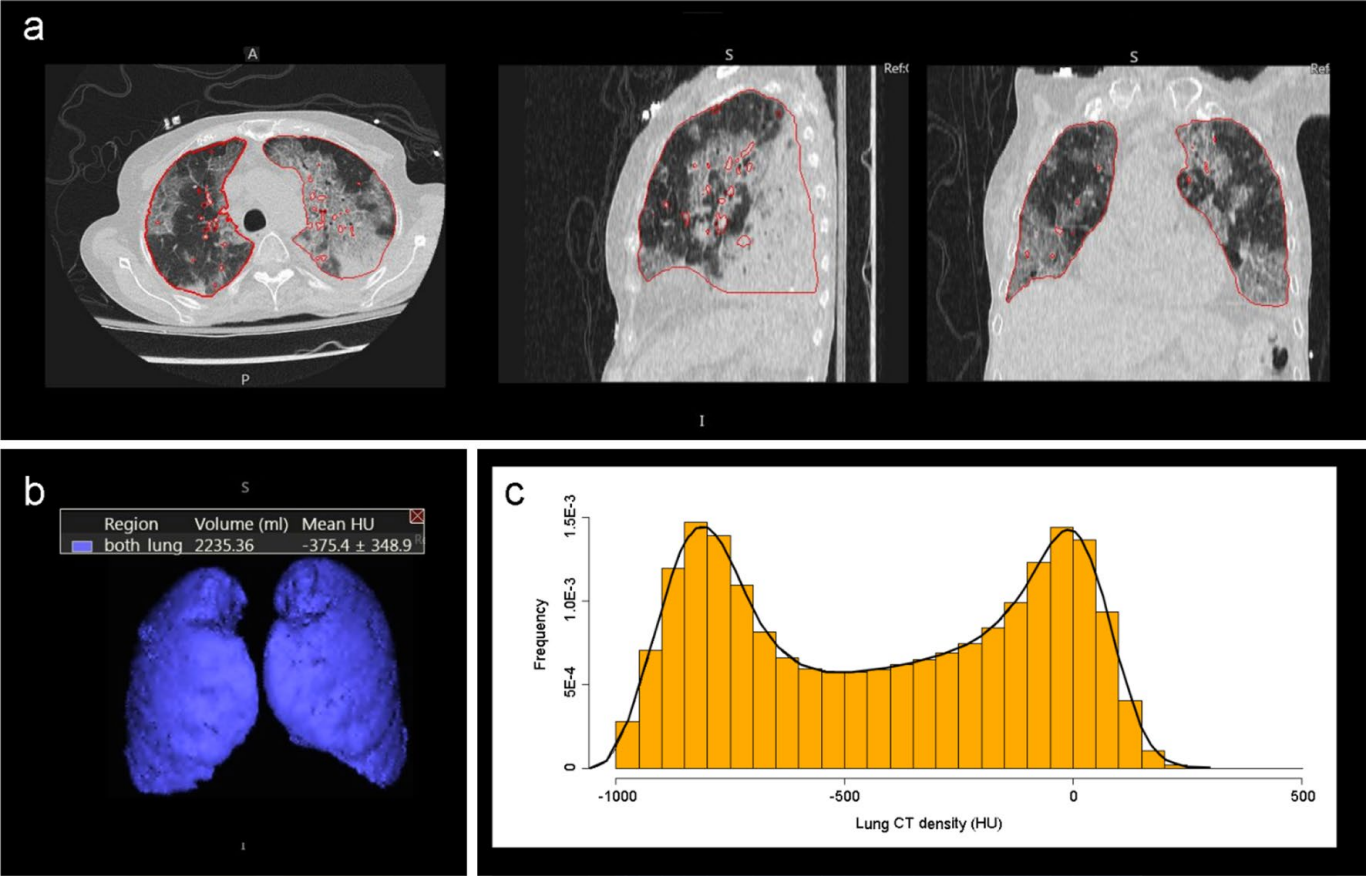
Non-contrast low dose chest CT images in a 62-year-old woman with severe COVID-19 pneumonia. The patient was admitted to ICU, required ventilation, and died in hospital. (a) CT images show bilateral extensive ground glass opacities and septal thickening. (b) 3D view with volume. (c) Histogram of CT density values demonstrates 2 peaks, the first peak at − 800 HU representing normal well aerated lung and the second peak at 0 HU representing the lung consolidations and ground glass opacities. Mean lung density: − 375.4 HU (98th percent.), SD-CT: 348.9 HU (94th perc.), skewness: − 0.02 (7th perc.), kurtosis: 1.53 (0.6th perc.), Q875: 36HU (88th perc.), F1: 0.77 (98th perc.), F2: − 0.33 (9th perc.).
